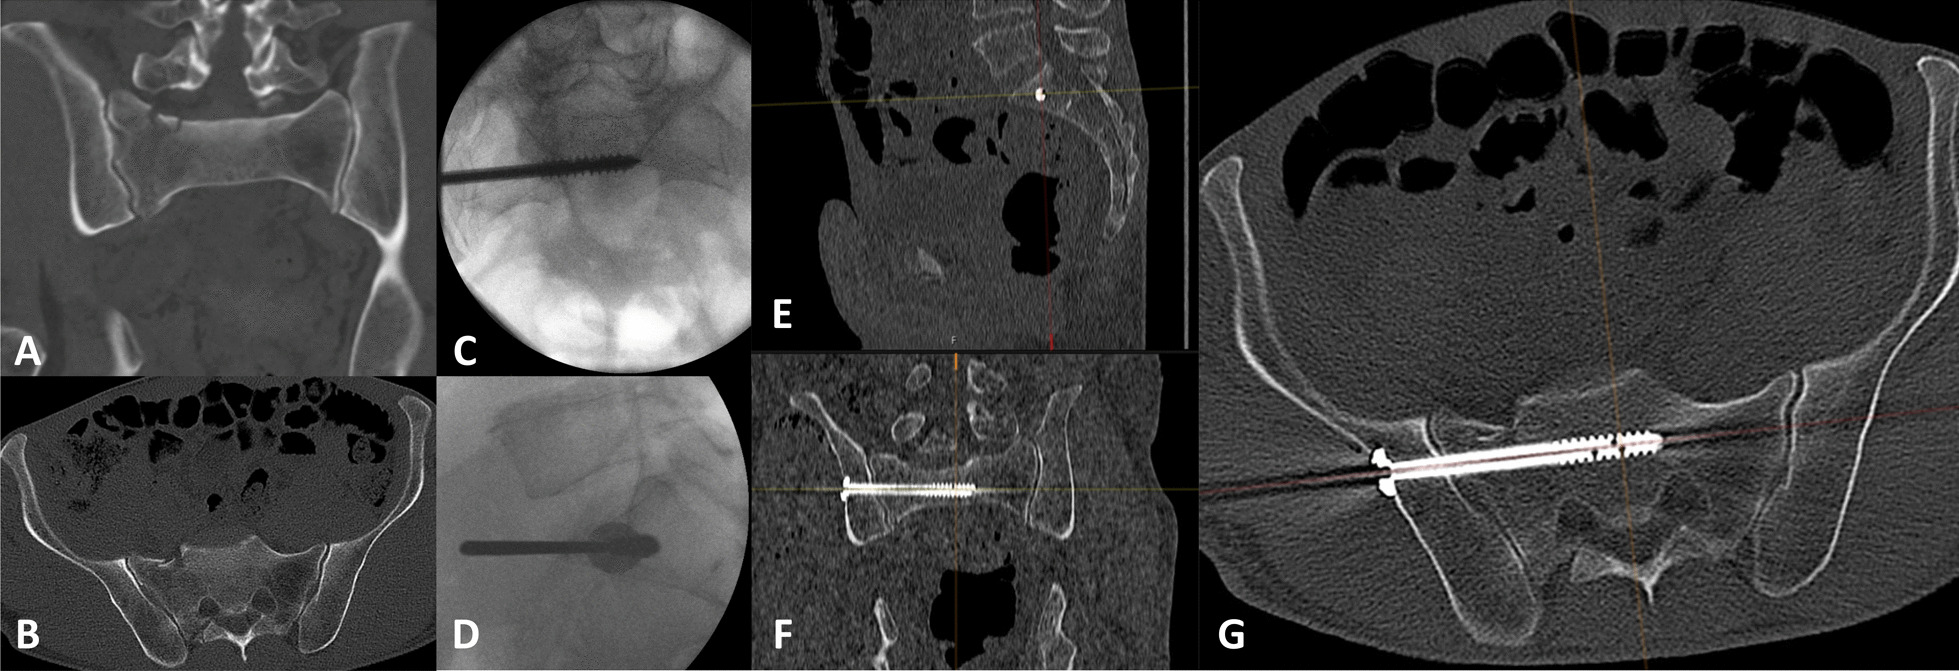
Exemplarily preoperative, intraoperative and postoperative radiographs of a patient treated by MIS ISS placement guided by navigation for a traumatic fracture of the sacrum. A and B shows the preoperative CT scan (A in frontal view, B in axial view). C and D shows p.a. (C) and lateral (D) intraoperative radiographs after the ISS placement guided by navigation with the guide wire still in situ. E–G shows screw positioning in the postoperative CT scan (E in sagittal, F in frontal view and G in axial view)
